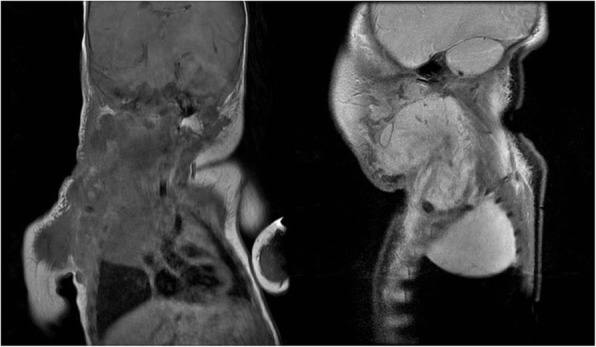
Case 3. Cervico-mediastinal lymphatic malformations after partial excision, visualized on magnetic resonance imaging (coronal and sagittal images)
